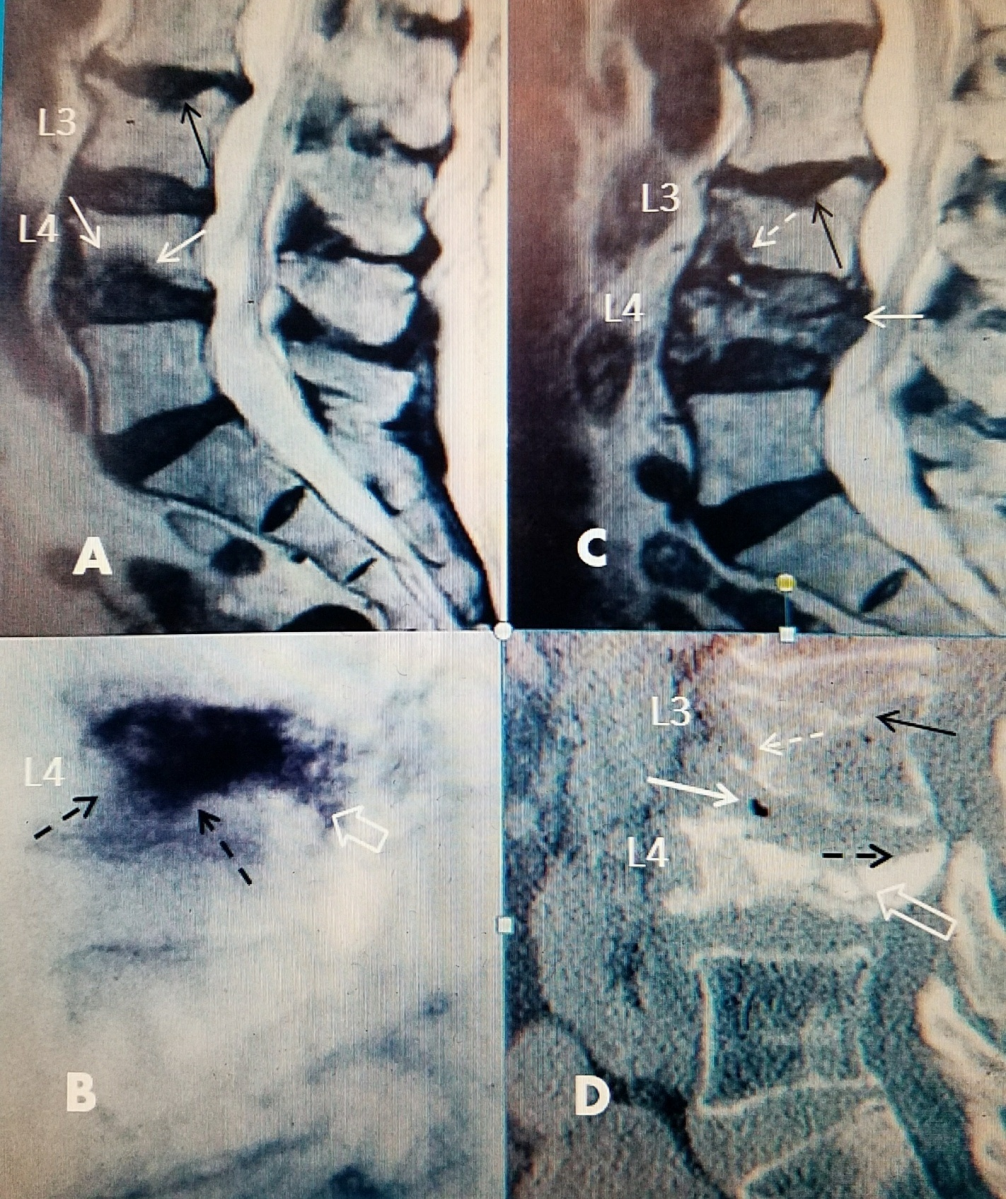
Progression of L4 inferior endplate fracture after vertebral augmentation A: Sagittal T2 magnetic resonance image (MRI) showing inferior anterior endplate edema at L4 (white arrows) and an old minimal superior endplate fracture at L3 (black arrow). B: Intra-operative films after bilateral vertebral augmentation showing diffuse spread of bone cement (empty white arrow). There is spread of bone cement under the entire superior endplate into inferior anterior vertebral body toward the fracture site (dotted black arrows). C: Four weeks later, there is progressive collapse of L4 now associated with protrusion of posterior wall of the vertebral body into the spinal canal (solid white arrow). At the same time, a new edematous change has developed in the adjacent vertebrae at the inferior anterior part of L3 (dotted white arrow). This was not present on the initial MRI scan. There is also the beginning of very slight kyphotic angulation at L3 over L4 that was not seen on the initial scan. D: Computerized tomography (CT) six weeks after the initial procedure showing further progressive collapse of L4 (hollow white arrow). There is also a new vacuum change in the anterior part of the L3-4 disc space (solid white arrow). There is developing collapse anterior inferior at the L3 vertebrae (dotted white arrow). The L3 superior endplate fracture is unchanged (solid black arrow).
